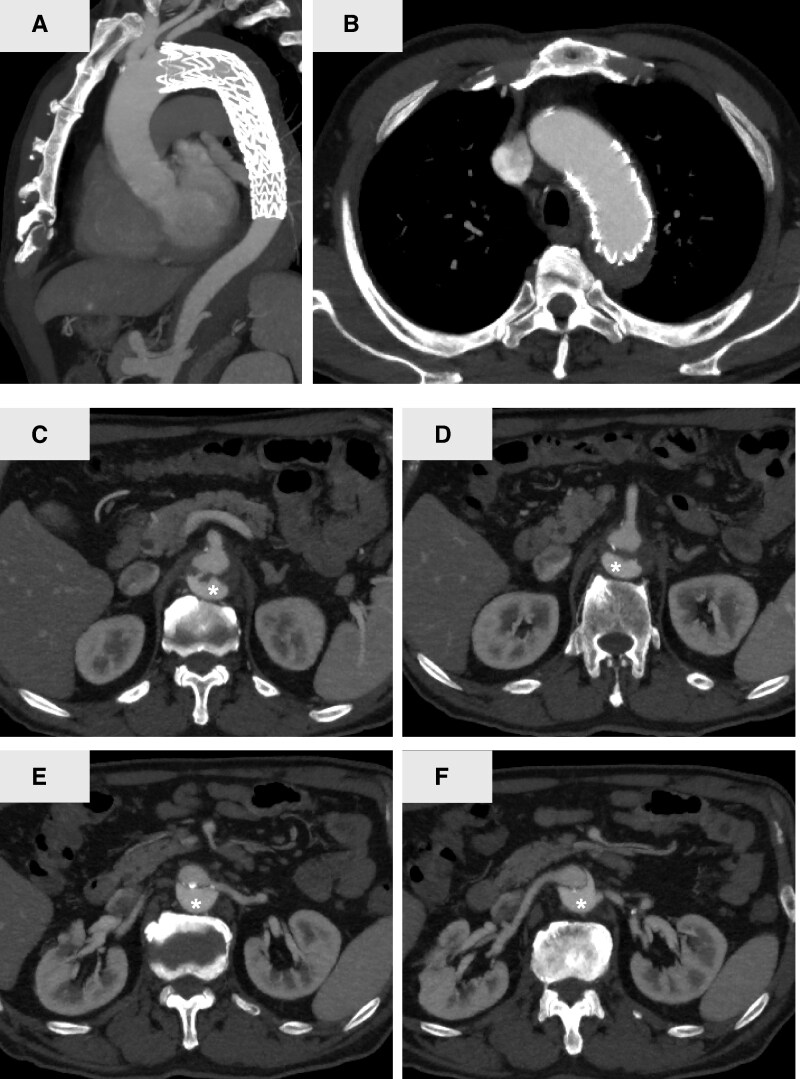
Enhanced computed tomography images at 14 days after thoracic endovascular aortic repair. Sagittal (*A*) and axial (*B*) images of the distal aortic arch. The celiac artery (*C*), superior mesenteric artery (*D*), left renal artery (*E*), and right renal artery (*F*) branch from the true lumen. The density at the kidney during the early phase is improved. An asterisk (*) indicates the false lumen.
